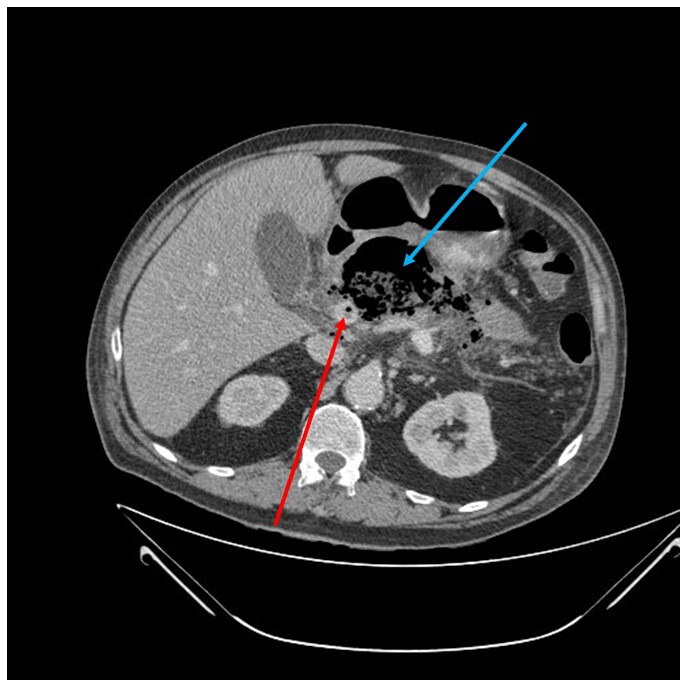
CT scan showing gas collection (blue arrow) and a thrombus inside the portal vein (red arrow). These findings characterize emphysematous pancreatitis and phylephlebitis.
